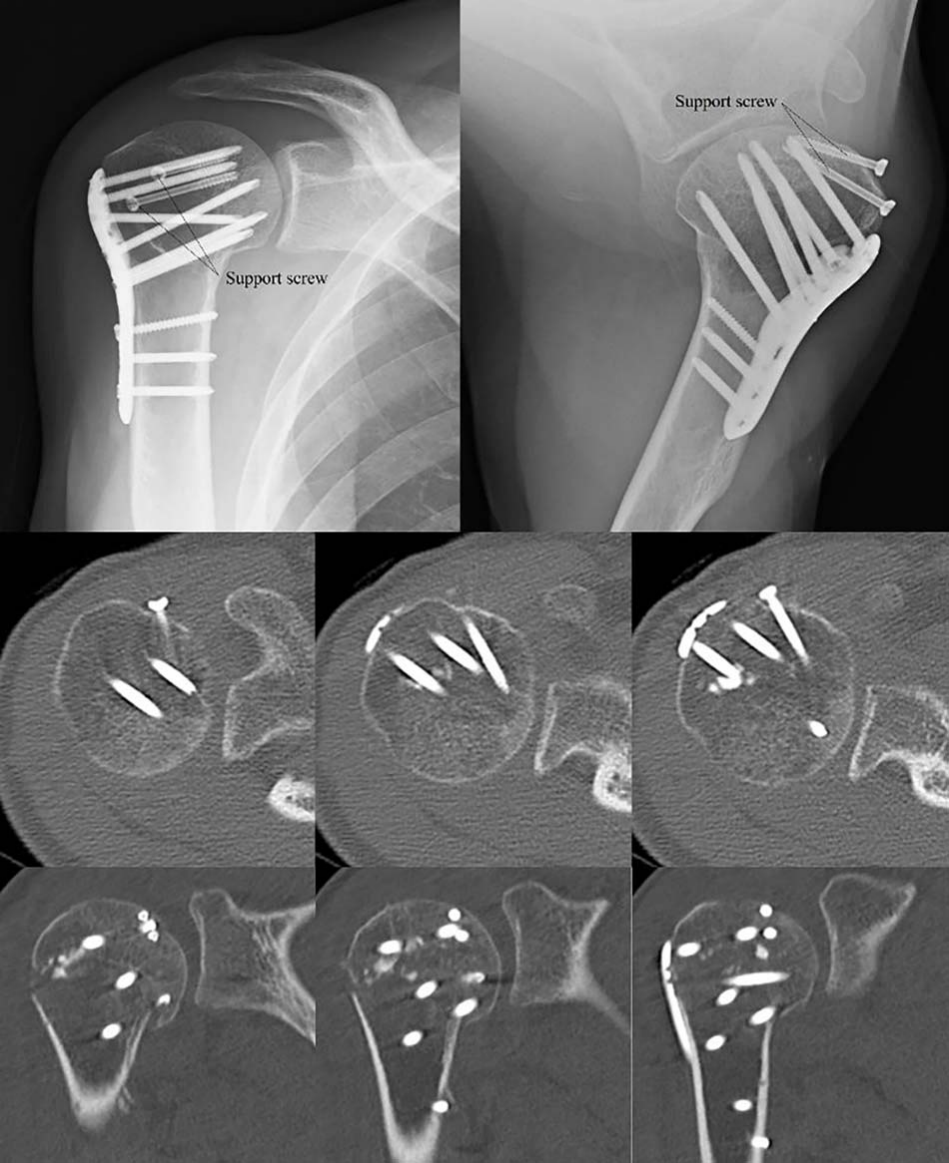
Postoperative X-ray and CT scan showing sustained anatomical reconstruction of articular surfaces and no signs of osteoarthritis.
